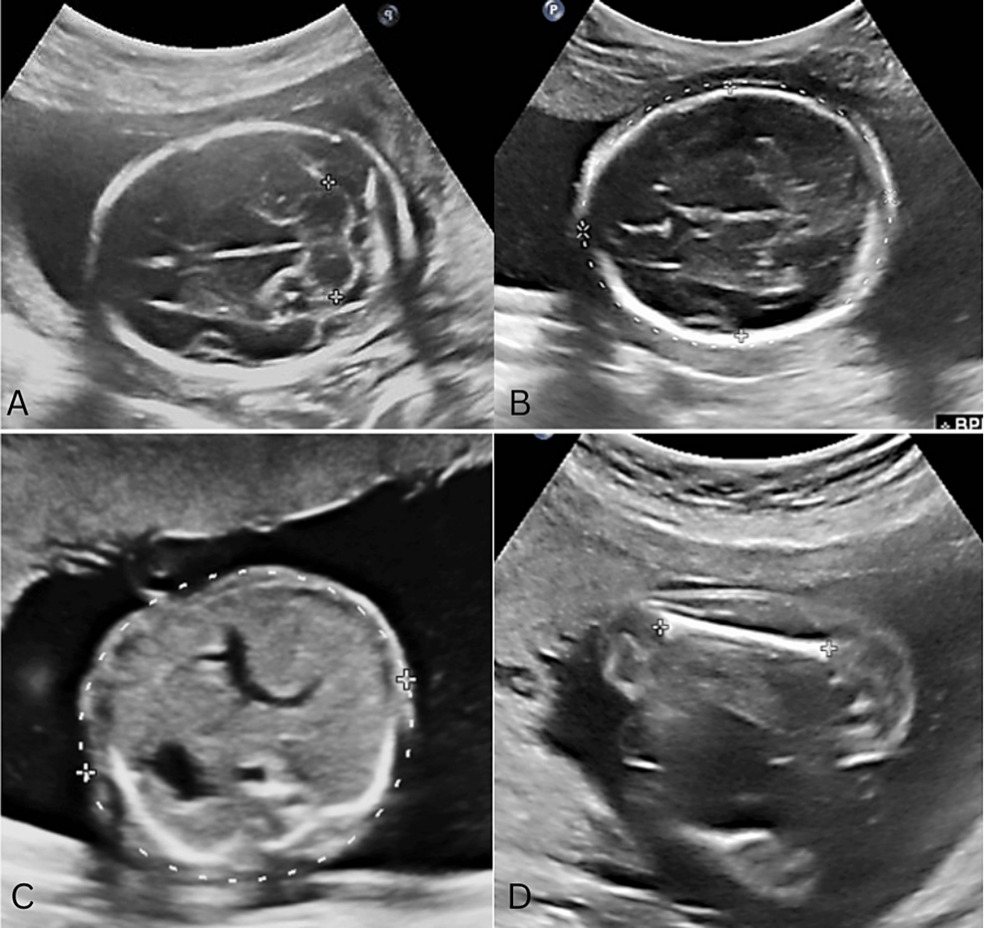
Fetal biometry. Ultrasonographic measurement of (A) TCD, (B) BPD and HC, (C) AC, and (D) FL TCD: transcerebellar diameter; BPD: biparietal diameter; HC: head circumference; AC: abdominal circumference; FL: femur length
